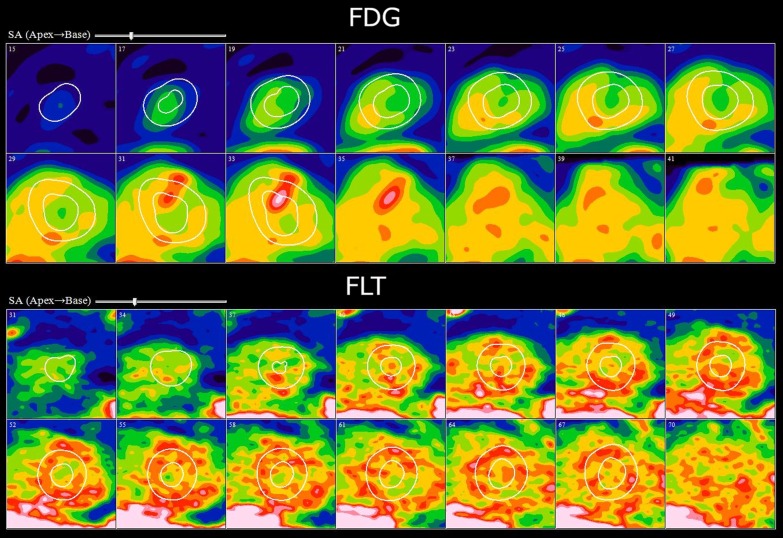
Cardiac short axis views of subject #10 demonstrating a focus of myocardial uptake in the basal anterior wall on FDG-PET (top) without corresponding uptake on the FLT-PET study (bottom). The diffuse heterogeneous uptake seen on the FLT study is in keeping with background blood pool uptake
